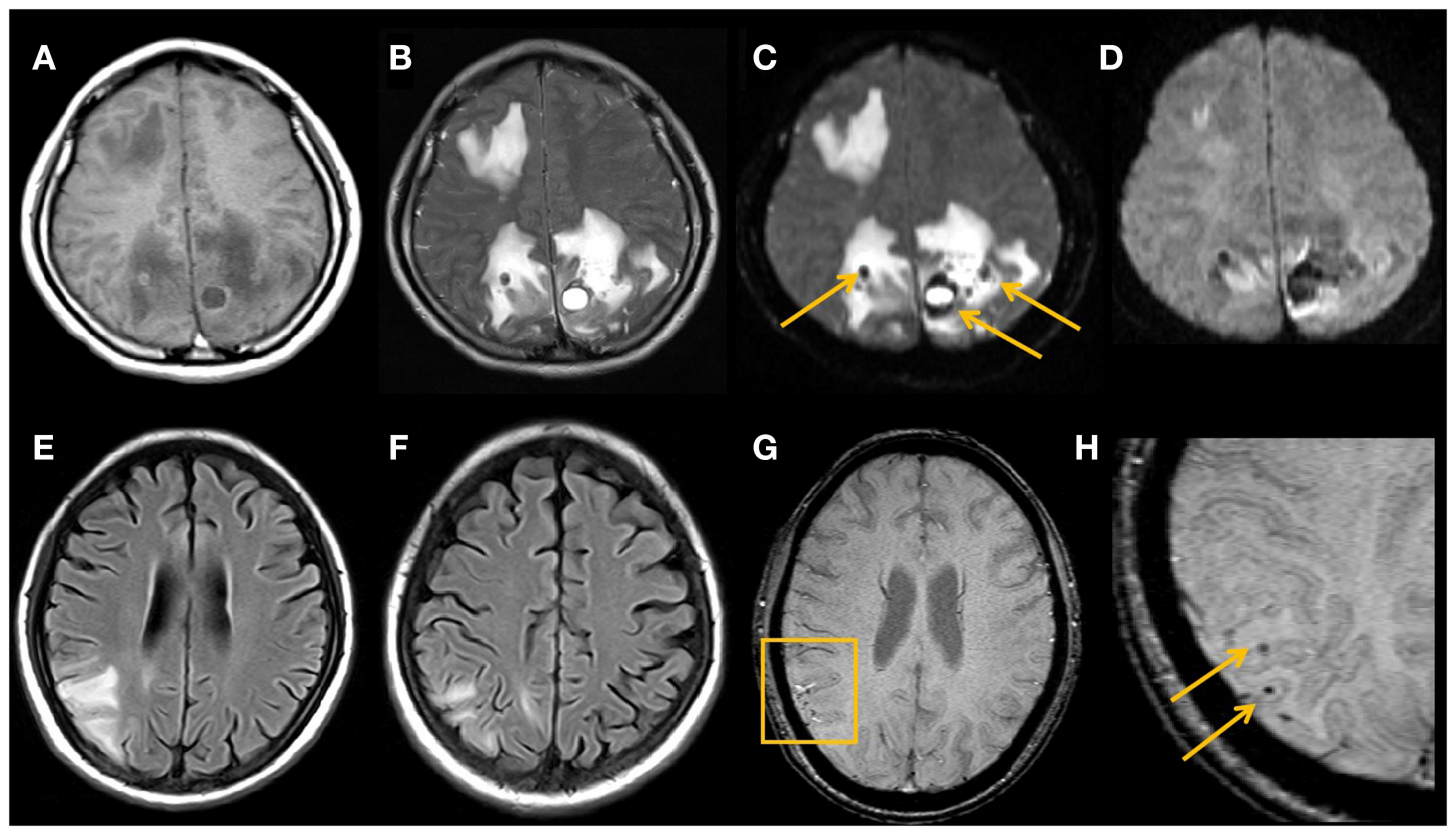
Typical MRI findings in hemorrhage secondary to PRES.Imaging studies in a 29-year-old female with lupus (A–D). MRI shows vasogenic edema in the right frontal lobe and bilateral parieto-occipital lobes, multiple hematomas are seen in the area affected by the edema, with heterogeneous high signal on T1WI (A), low signal on T2WI (B), low signal on DWI b = 0 images (arrows) (C), and magnetically sensitive artifacts of high signal around the hematoma visible on DWI b = 1,000 images (D). An 18-year-old young female with nephrotic syndrome (E–H). PRES-related right parieto-occipital cortical and subcortical edema on MRI FLAIR image (E,F), with multiple small microhemorrhages on SWI (arrows) (G,H).
